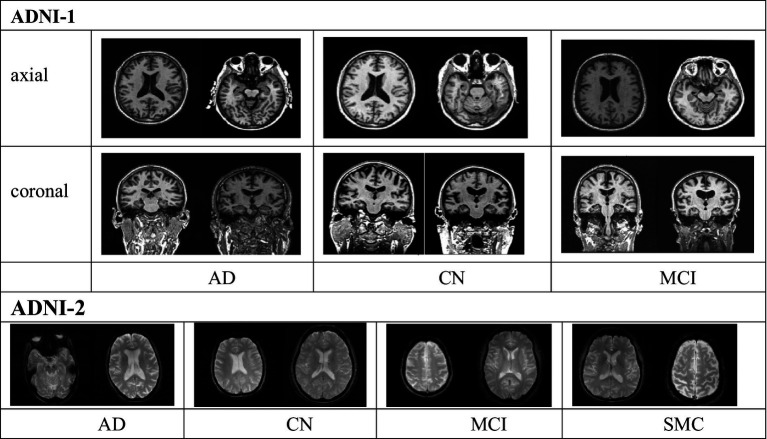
The MRI samples of the ADNI datasets.
